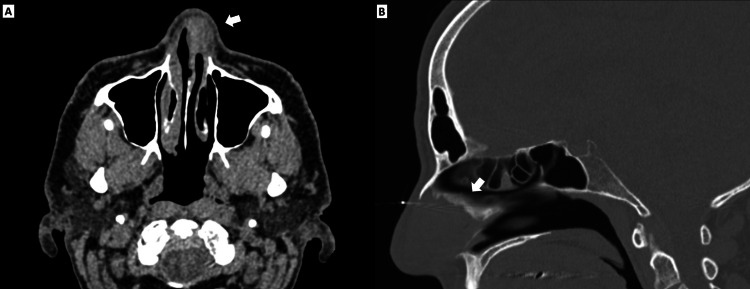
Contrast-enhanced transverse (A) and sagittal CT sections (B). Maxillofacial CT scan noting mass in left anterior nasal vestibule measuring 1.5 × 1.6 × 1.4 cm.
